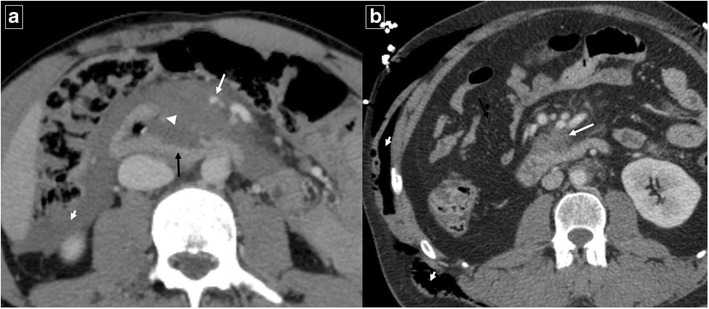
Duodenal trauma in different patients. a A 20-year-old man with blunt abdominal trauma. Contrast-enhanced CT shows an extensive pancreaticoduodenal hematoma with displacement of the mesenteric vessels to the left (white arrow) and disruption of the wall of the third duodenal portion (black arrow) with active extravasation of intravenous contrast (arrowhead). Note the abundant retroperitoneal fluid (short arrow). b A 45-year-old man involved in a motor vehicle accident. Contrast-enhanced CT shows free retroperitoneal fluid (arrow) adjacent to the third duodenal portion and slight wall thickening or mural edema. Abdominal subcutaneous emphysema (short arrows) secondary to multiple rib fractures and pneumothorax
